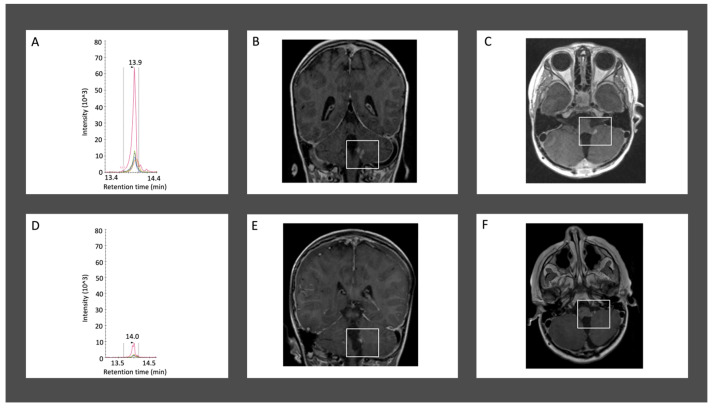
Longitudinal multimodal surveillance demonstrating concordant reduction in CSF endogenous light signal and interval improvement in MRI findings in a treated medulloblastoma patient (1659). (A–C) correspond to the first post-treatment surveillance timepoint (June 2020) and (D–F) correspond to the second surveillance timepoint (August 2020). (A) Endogenous light signal chromatogram for peptide biomarker SSNSPHSPIVEEQVPYNK from the June 2020 lumbar puncture, showing a prominent peak. (B,C) Coronal (B) and axial (C) T1-weighted MRI with contrast demonstrating an enhancing nodule within the left cerebellomedullary fissure (box) at the same June 2020 timepoint. (D) Chromatogram from the August 2020 lumbar puncture demonstrating a sharp decline in endogenous light signal for the same peptide marker. (E,F) Coronal (E) and axial (F) T1-weighted MRI from August 2020 showing interval reduction in nodularity (box) at the previously visualized site.
